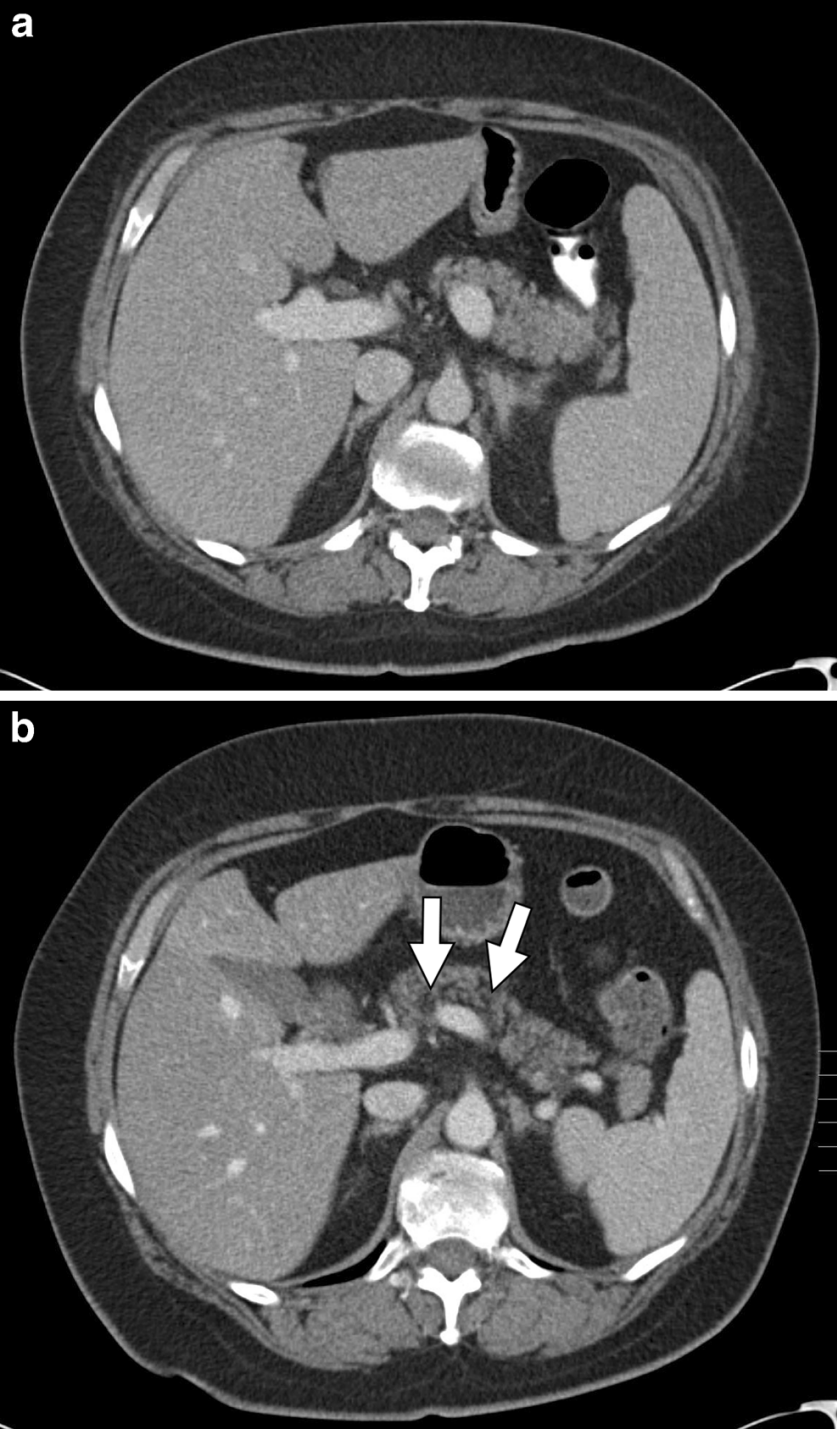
A 57-year-old female with leimyosarcoma treated with ifosfamide, mesna, and doxorubicin. a Pre-chemotherapy axial contrast-enhanced CT shows minimal fatty replacement of the pancreas. b Diffuse fatty replacement of the pancreas (*arrows*) is noted on axial contrast-enhanced CT performed 3 years later following cessation of chemotherapy
